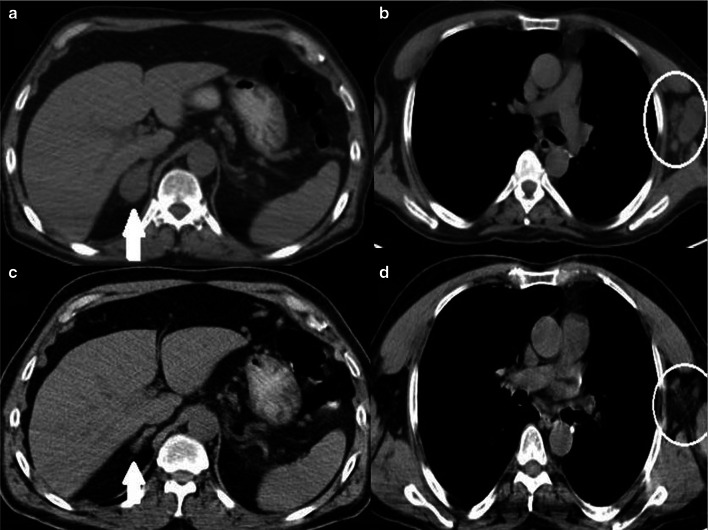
Complete response, a 74-year old male patient with metastatic non-small cell lung cancer under treatment with nivolumab (anti-PD-1 monoclonal antibody). a, b Axial CT images show metastasis in the right adrenal gland (arrow) and multiple metastatic lymph nodes in the left axilla (circle). c, d There are no lesions higher than > 10 mm in both regions in the control images performed eight weeks after treatment initiation
